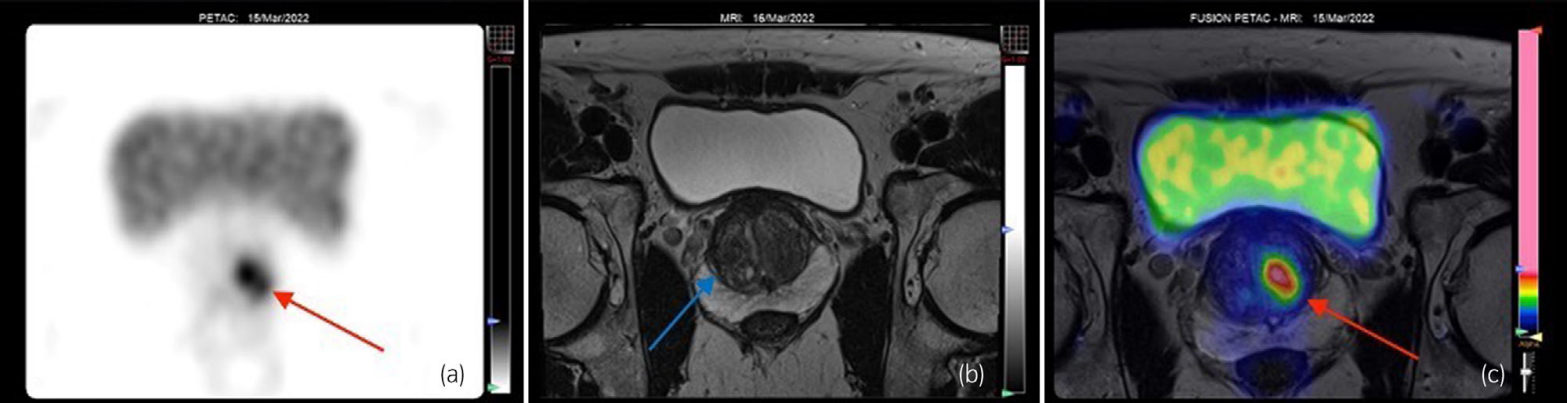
(a) 18F‐PSMA‐1007 PET scan showing left prostatic base activity (red arrow) and none on the right. (b) mpMRI was reported to be PIRADS 2 (blue arrow directed at right prostatic bed where GGG 4 biopsy was taken from). (c) Offline PSMA PET MRI fusion using semi‐automated fusion software identifying increased activity on left base (red arrow).
